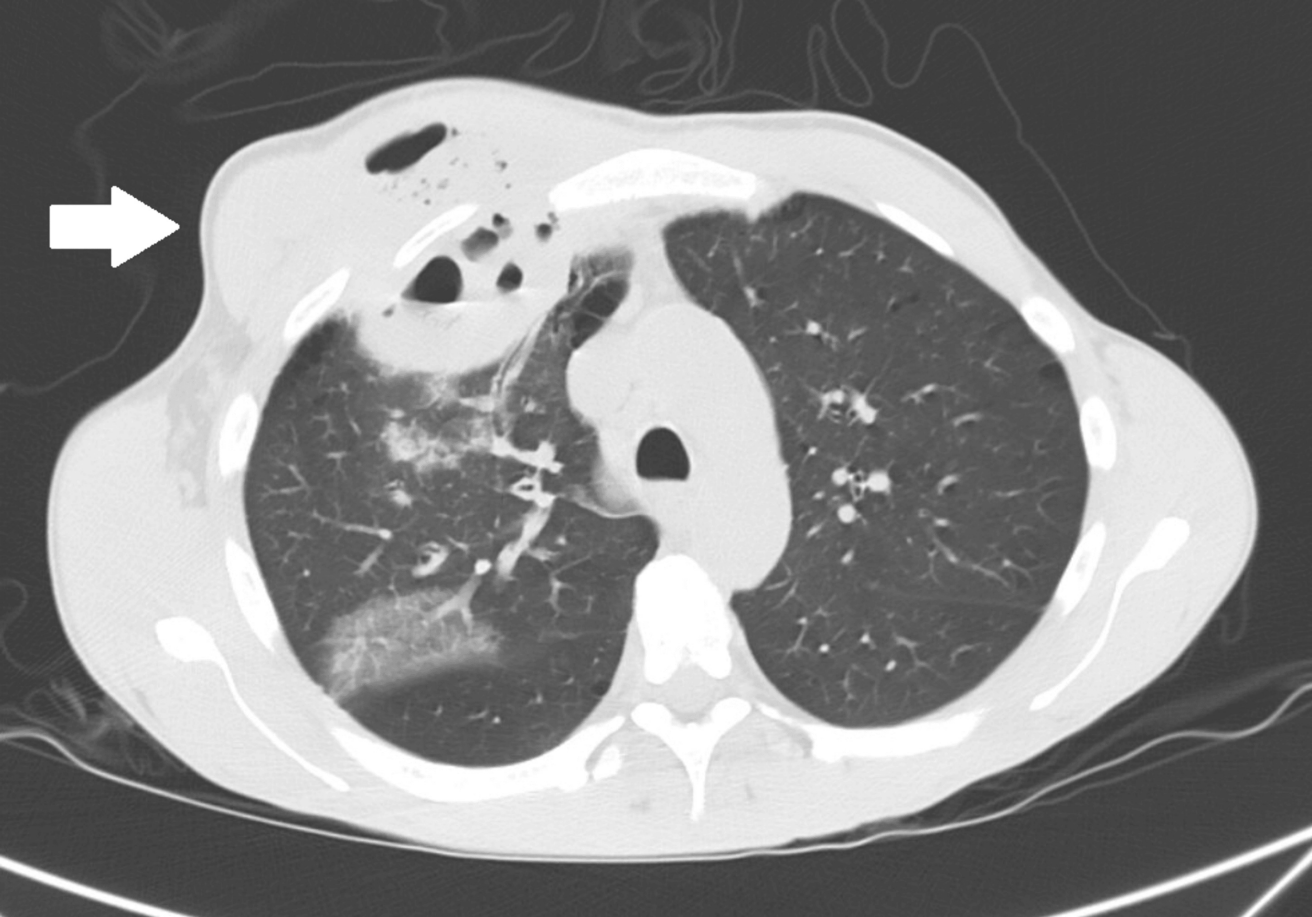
Axial chest CT (lung window) showing a cavitary lesion with internal gas in the right upper lobe, associated with surrounding inflammatory changes, confirming the pulmonary origin of the abscess.
